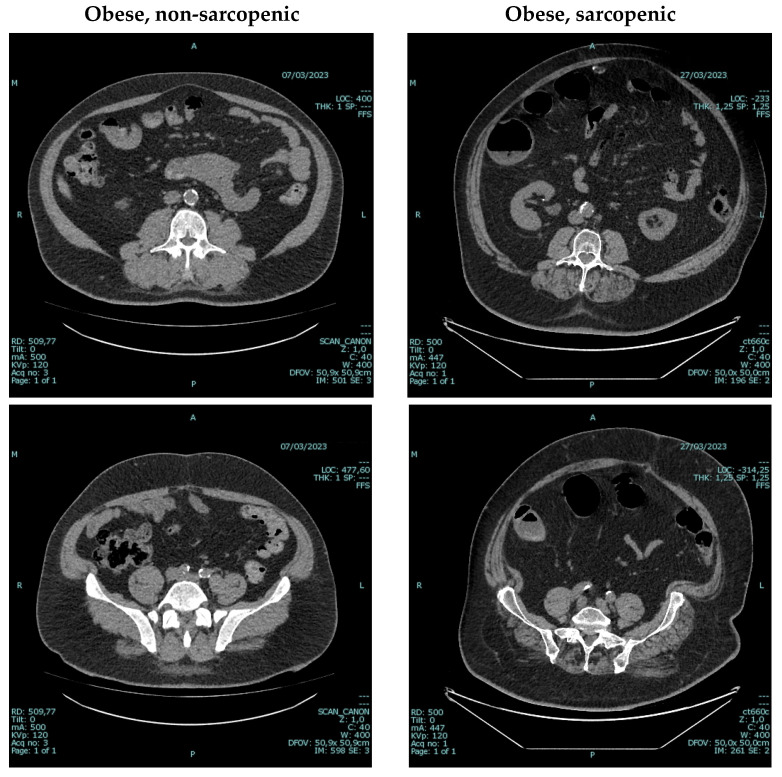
CT scan for the diagnosis of sarcopenic obesity. Legend: in addition to being used as traditional diagnostic tests, CT scans performed for other reasons provide valuable insights into the muscle mass of people living with CKD [81,82,83]. Free software exists to help interpret cross-sectional CT images, usually at the third lumbar vertebra (L3), or at the iliac crest, which can provide reliable estimates of body composition [84]. Notably, in the panel on the right, the quality and not only the quantity of muscle is impaired.
